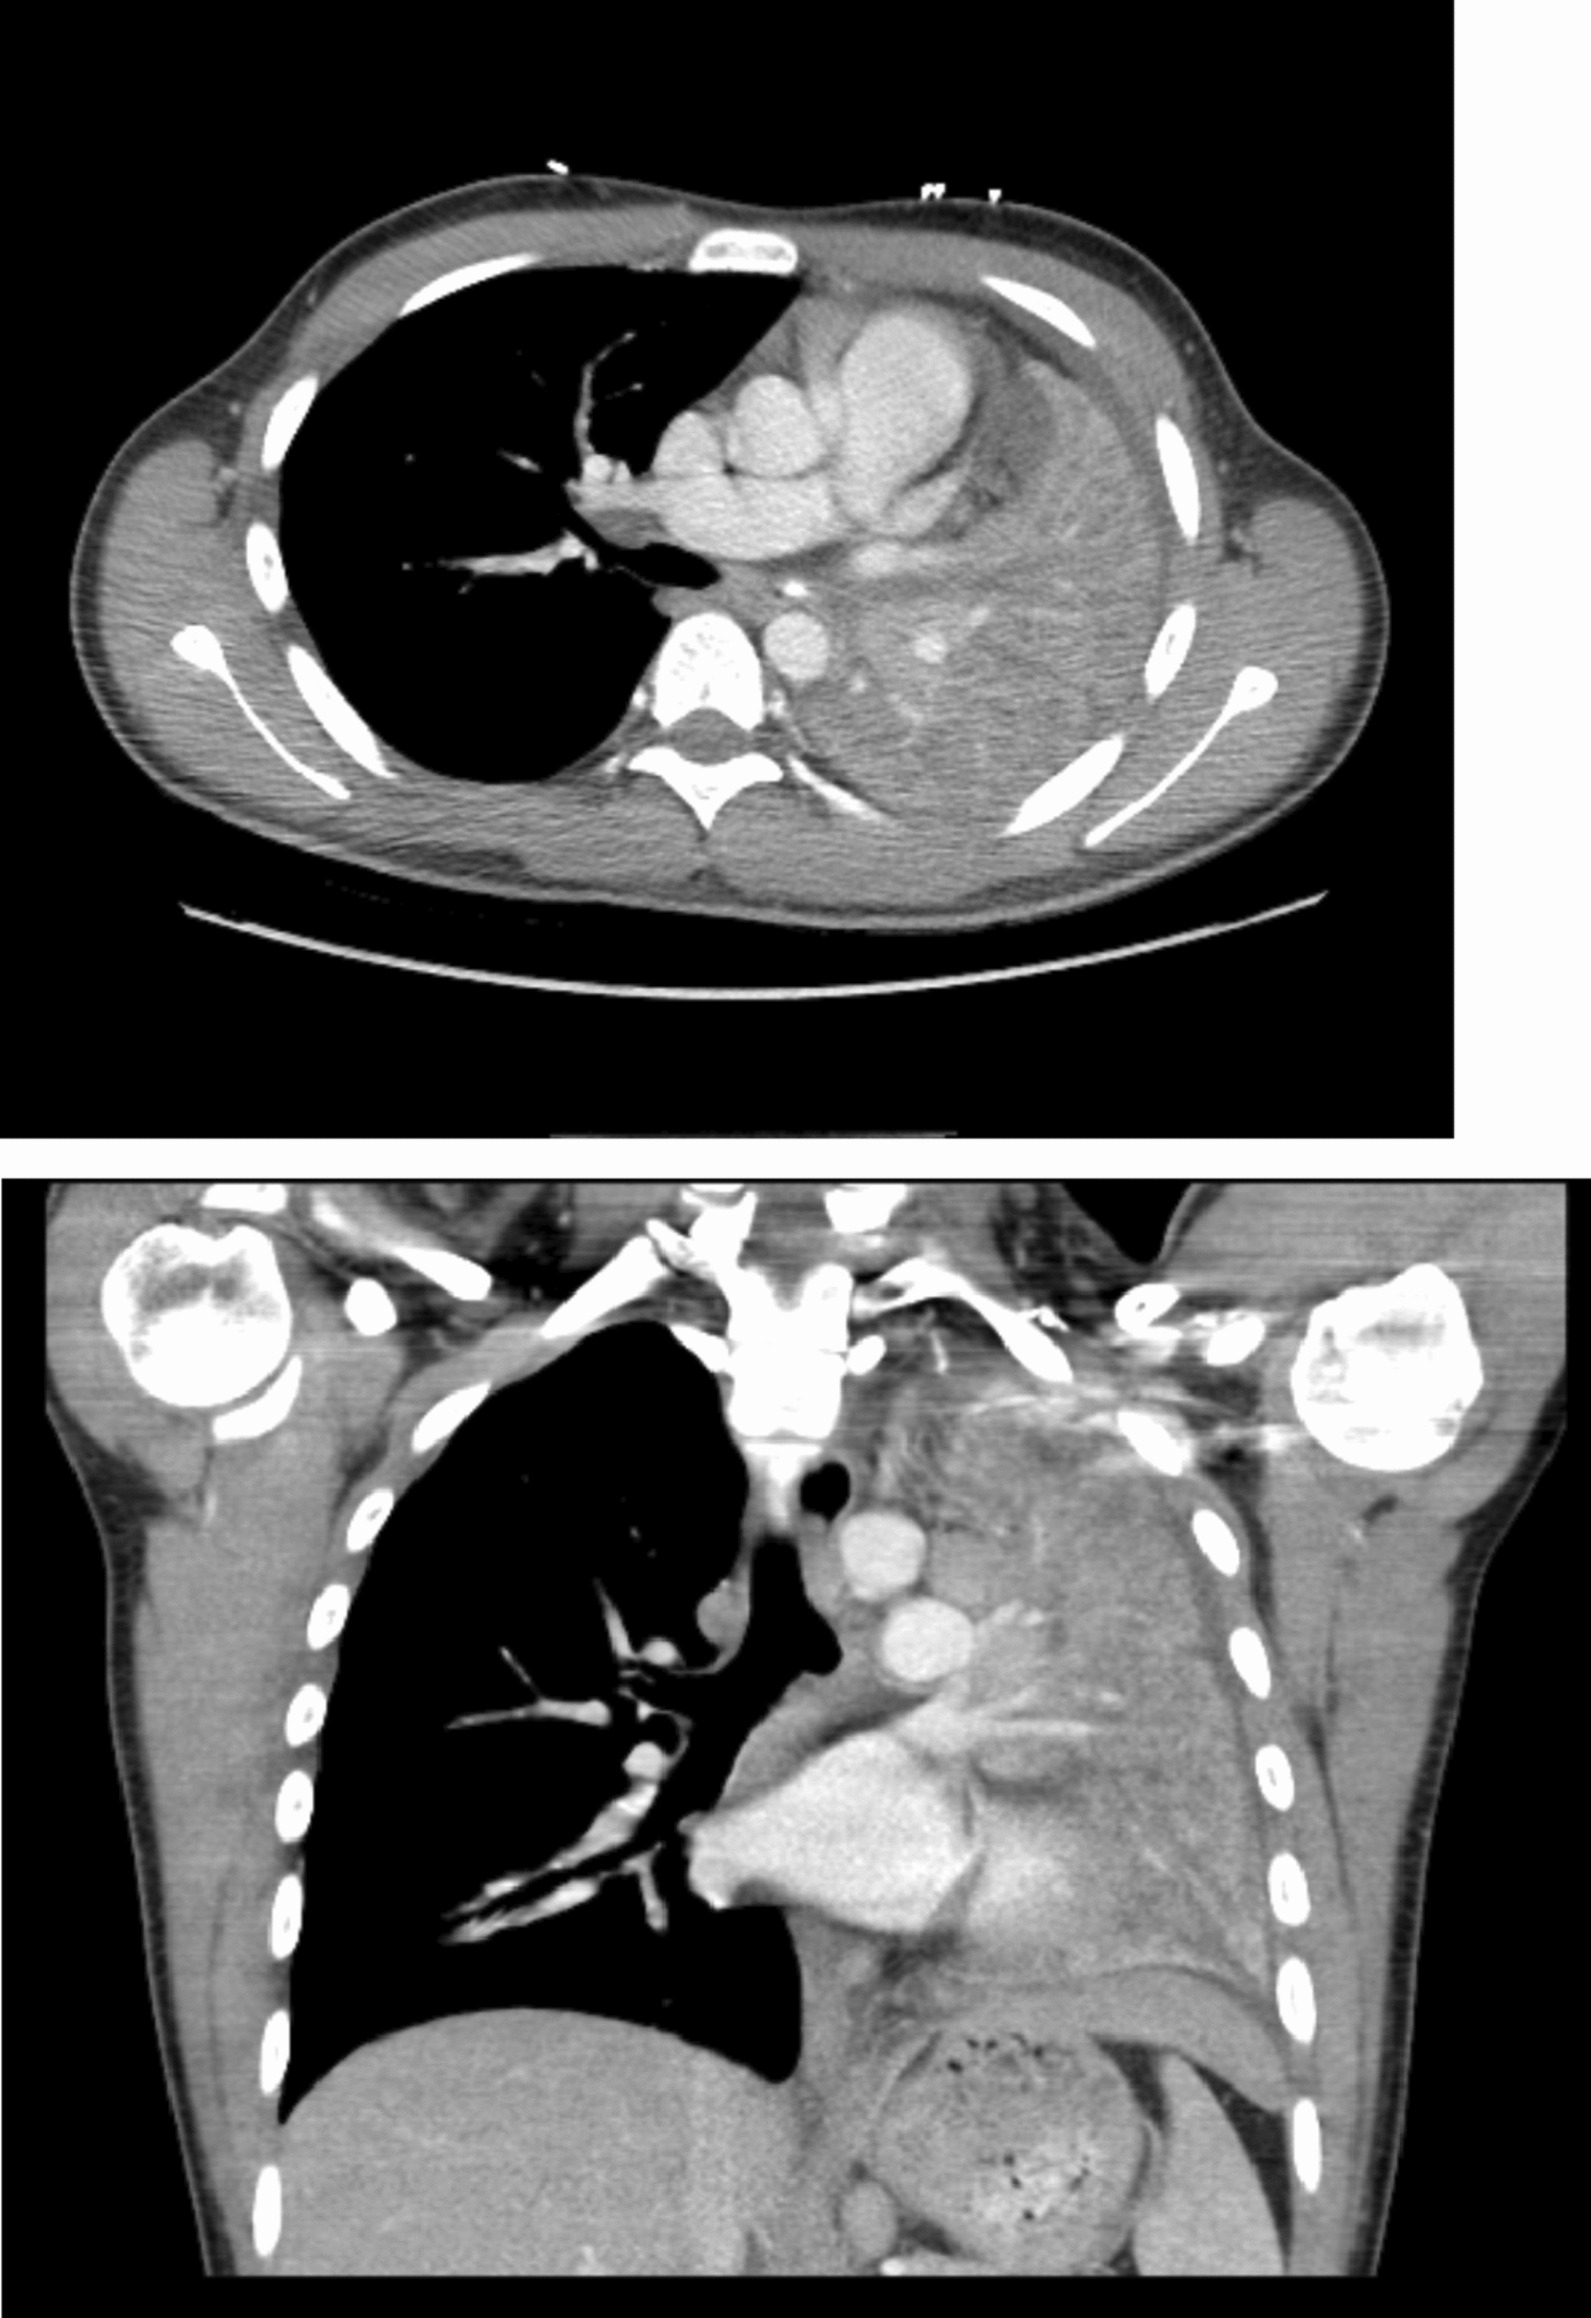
CT showing left bronchial obstruction secondary to tumour compression at presentation
